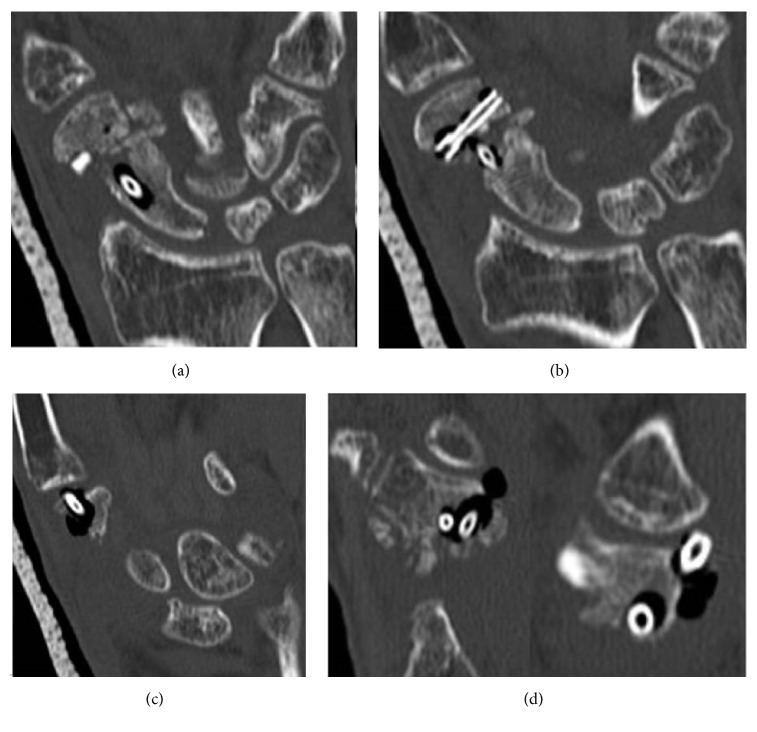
CT-scan taken 6 weeks after triscaphoid arthrodesis.
